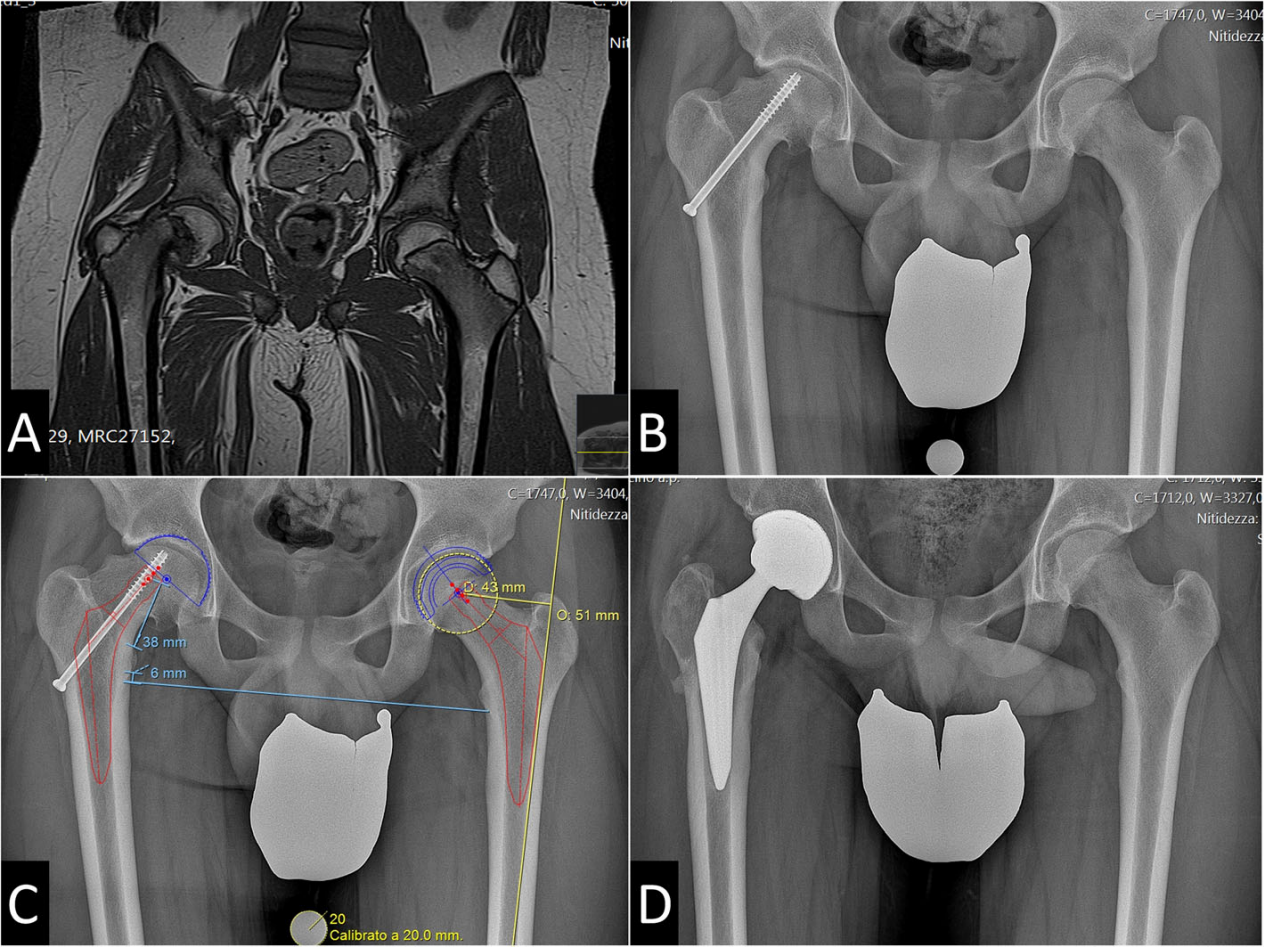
a Plain radiographs of a 15-year-old male with right SCFE (patient 5). b Screw fixation of the right hip. c Preoperative planning. d Postoperative radiograph after right THA using anterior approach
